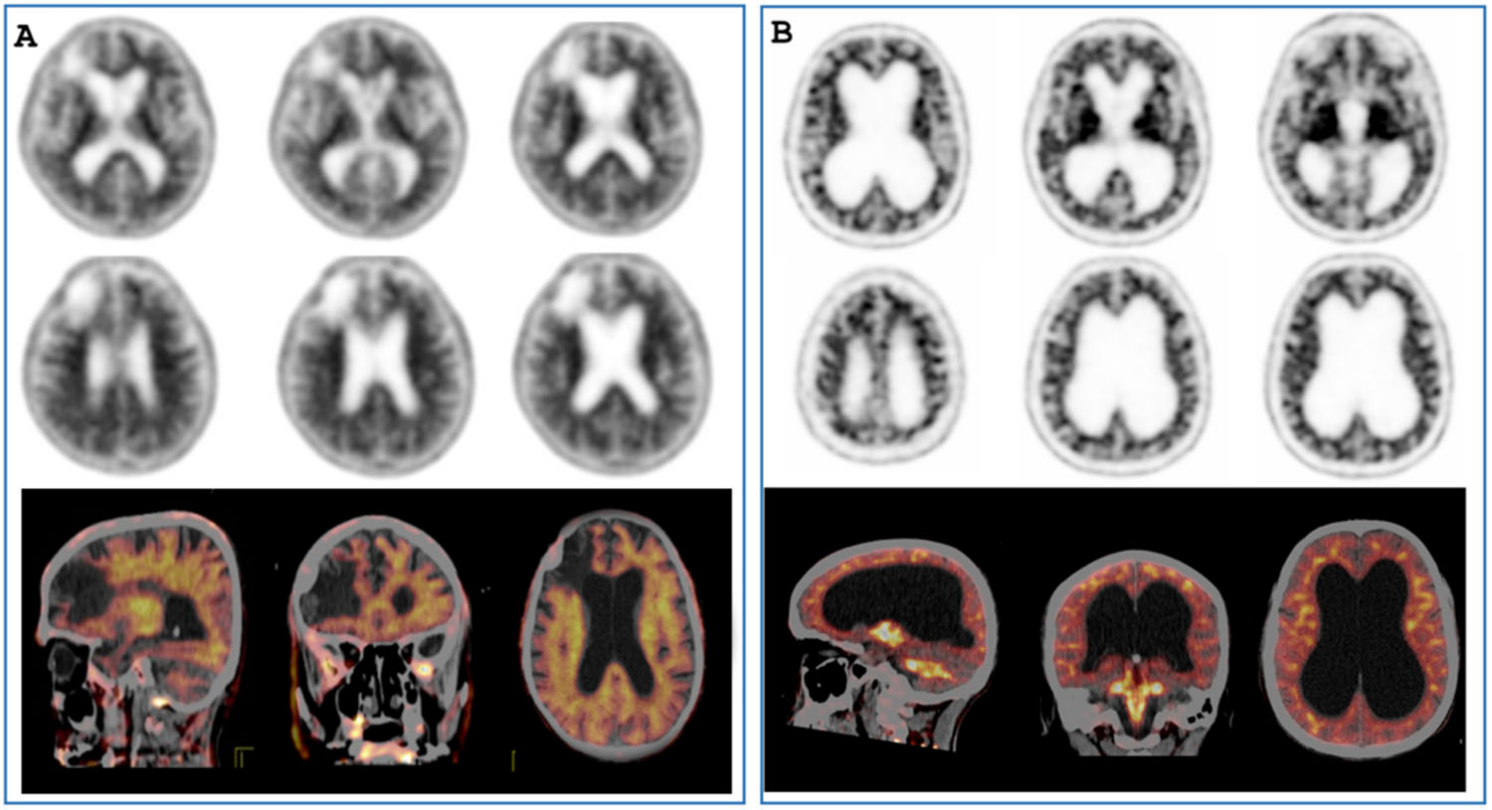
Pitfalls and artefacts in amyloid PET imaging. A [18F]Florbetapir PET/CT scan of a patient with right frontal stroke and brain atrophy. The PET/CT fusion images clearly show no difference between white matter and grey matter (positive amyloid PET scan). B [18F]Florbetapir PET/CT scan of a patient with normal pressure hydrocephalus. In this case, the fusion images showed much higher activity in the white matter than in the grey matter (negative amyloid PET scan)
